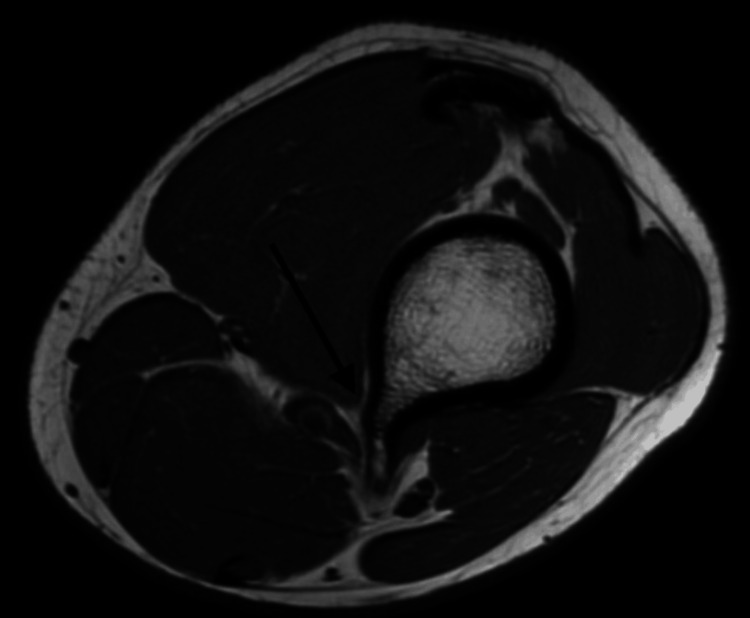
MRI T1-weighted axial imaging showing osteochondromas and their relation to the femur and neurovascular structures
